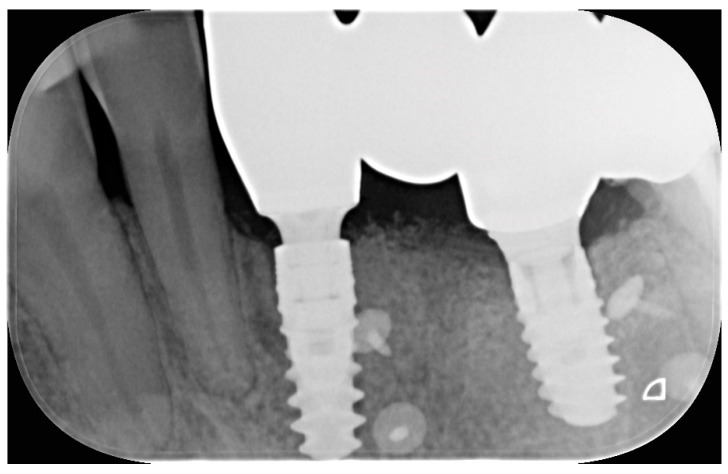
A peri-apical radiograph showing one of the included rehabilitations.
